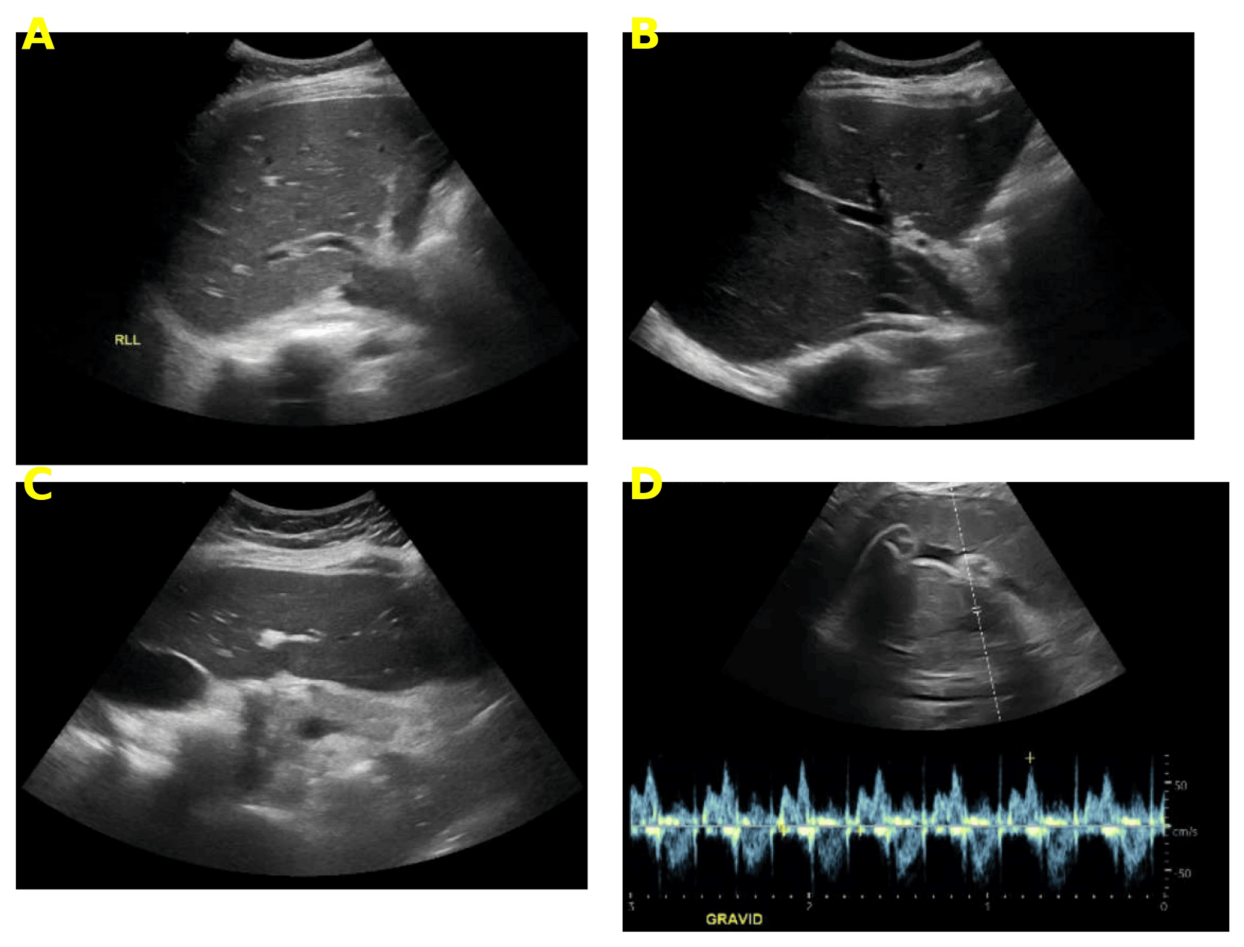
Abdominal ultrasonographic (A) Liver with homogeneous echotexture and smooth contour. No intrahepatic focal lesions or free peritoneal fluid are identified. (B) Longitudinal sonographic view of the gallbladder and biliary tree. The gallbladder appears normal with no wall thickening, calculi, or pericholecystic fluid. The common bile duct is not dilated. (C) Epigastric ultrasound with partial visualization of the pancreas, which appears hyperechoic and without focal lesions. (D) Transabdominal obstetric ultrasound confirms a viable intrauterine pregnancy with a Doppler waveform demonstrating fetal cardiac activity.
